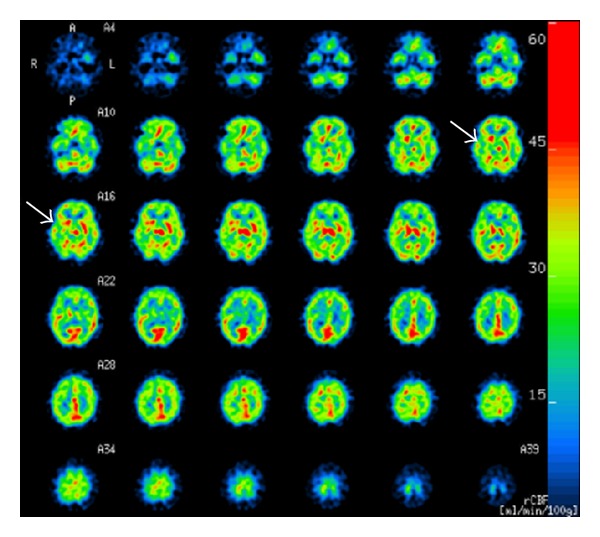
Brain perfusion scintigraphy (^123^I-IMP) showed a decrease in blood flow in the right lentiform nucleus (arrows) and bilateral symmetrical decreases in blood flow in the frontal, parietal, and temporal lobes.
